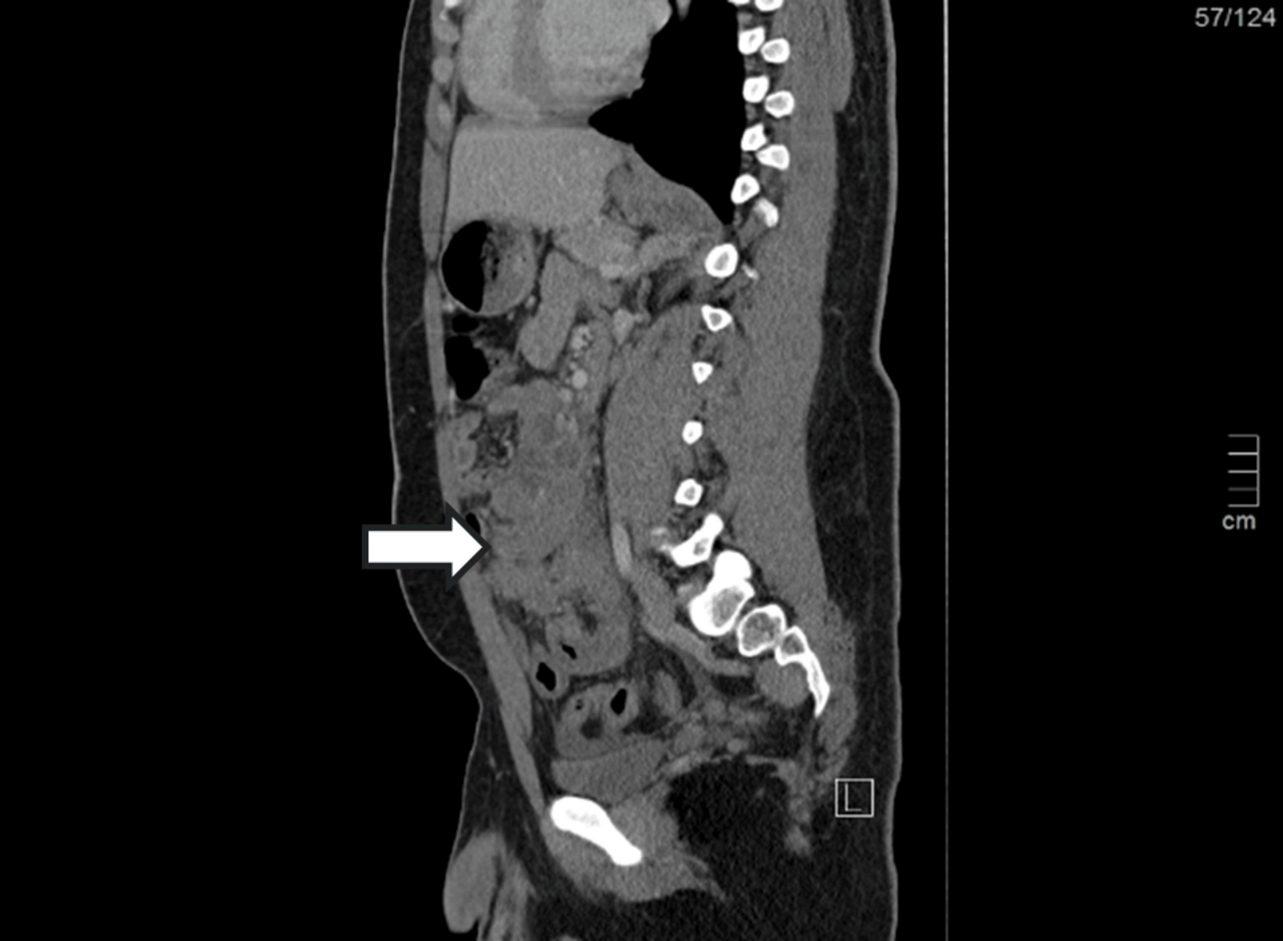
Abdominopelvic CT showing adenopathic conglomerate (side view)
